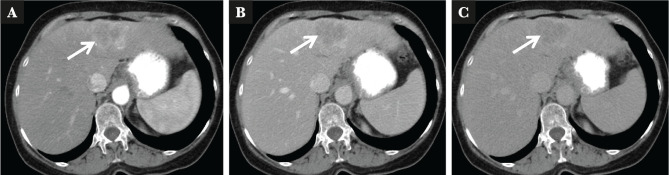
CT abdomen revealing a 3 cm lesion in the left liver lobe A – arterial phase; B – venous phase; C – portal phase.
